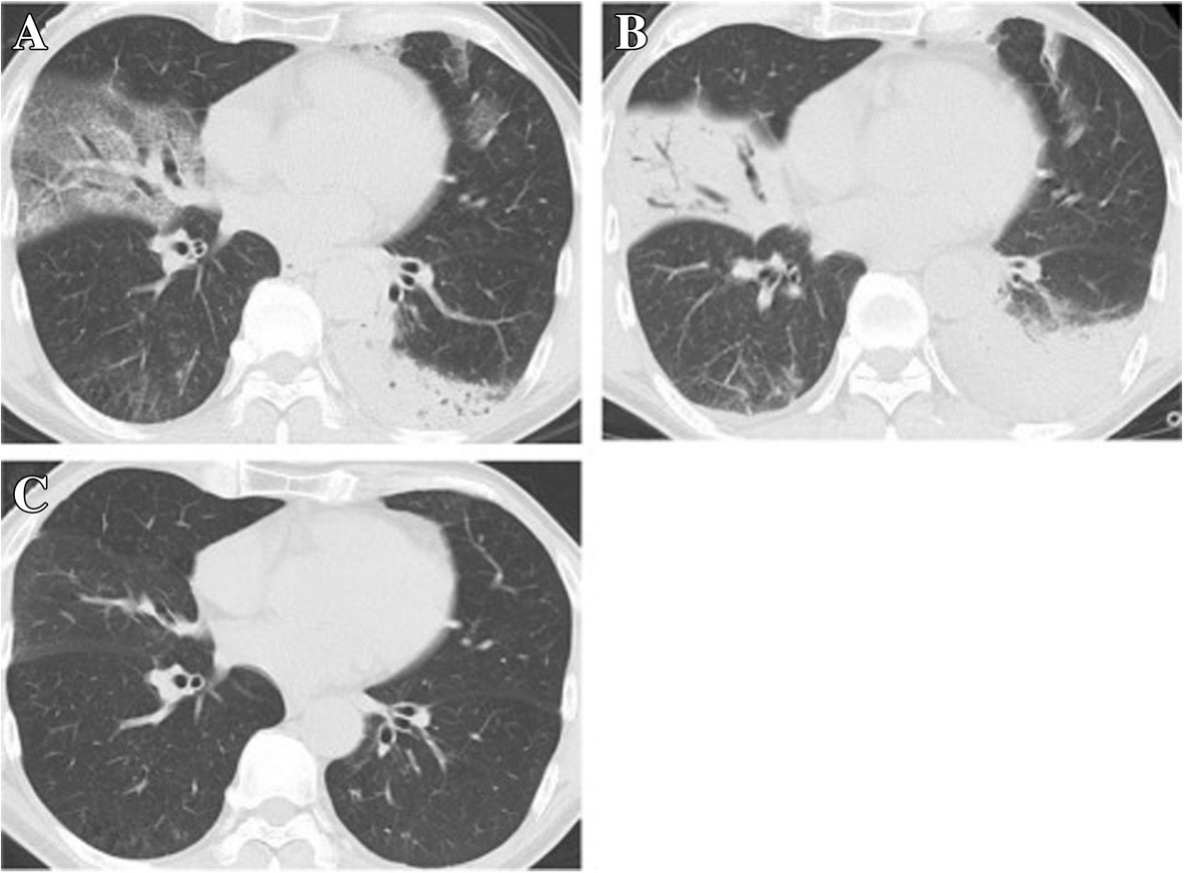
Typical CT images of non-relapse case. a Ground-glass attenuation with traction bronchiectasis in the right middle lobe and consolidation in the left lower lobe were recognized at the first visit. b Ground-glass attenuation changed into consolidation in the right middle lobe and consolidation apparently expanded in the left lower lobe just before treatment. c All shadows completely vanished after three months and relapse didn’t occur after one year
